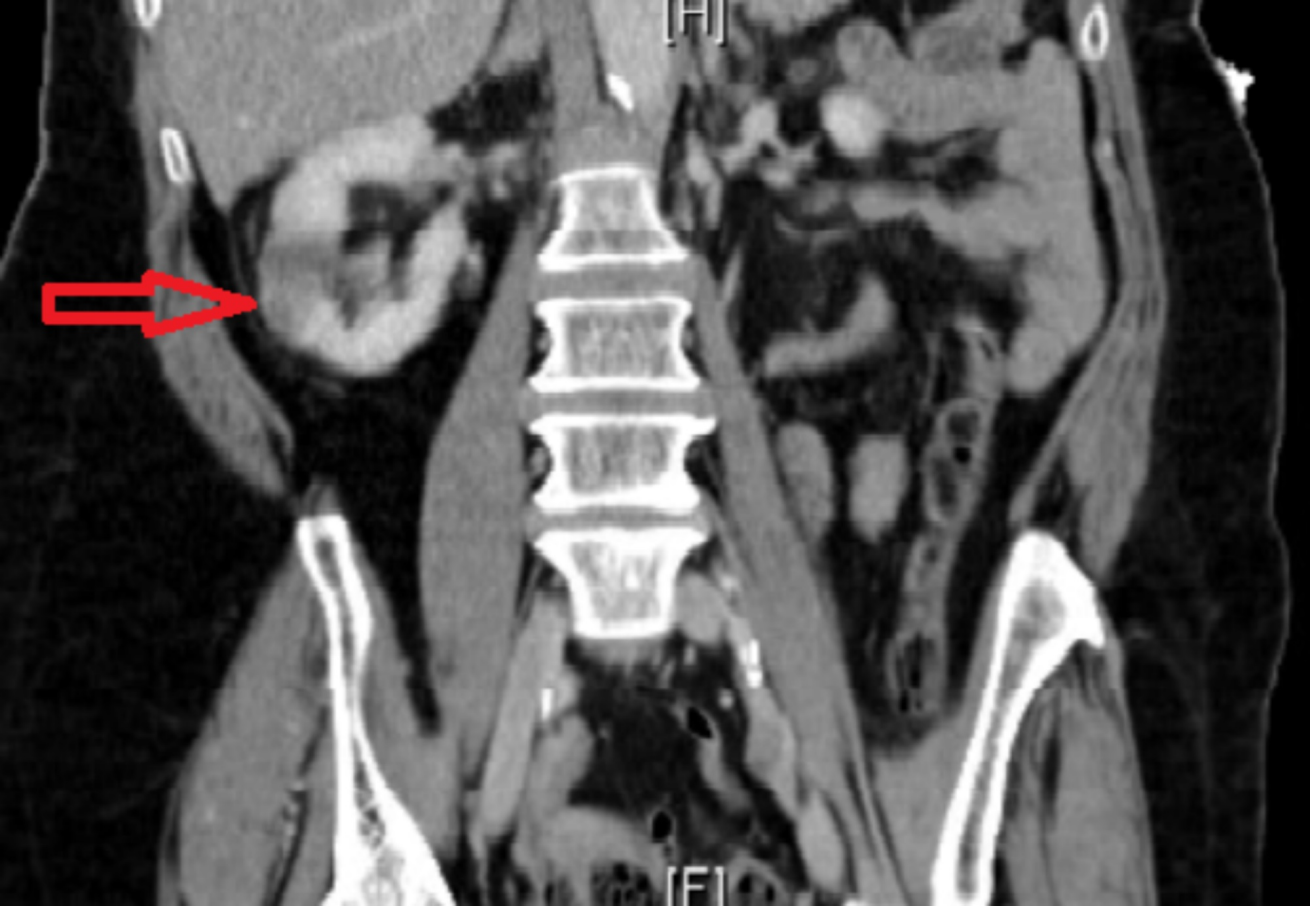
Computed tomography (CT) scan of the abdomen showing right renal infarct
